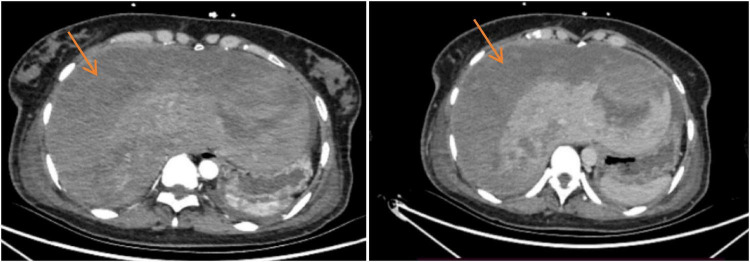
Admission contrast-enhanced abdominal CT. Arterial-phase contrast-enhanced abdominal CT obtained on admission demonstrated a large mixed-attenuation subcapsular hematoma in the right hepatic lobe with capsular bulging (arrow). A focal hepatic parenchymal laceration and punctate/patchy contrast extravasation were also identified, indicating active hepatic bleeding. Associated perihepatic fluid and abdominopelvic fluid were present, supporting the diagnosis of subcapsular hepatic hematoma with hepatic rupture and hemorrhage.
